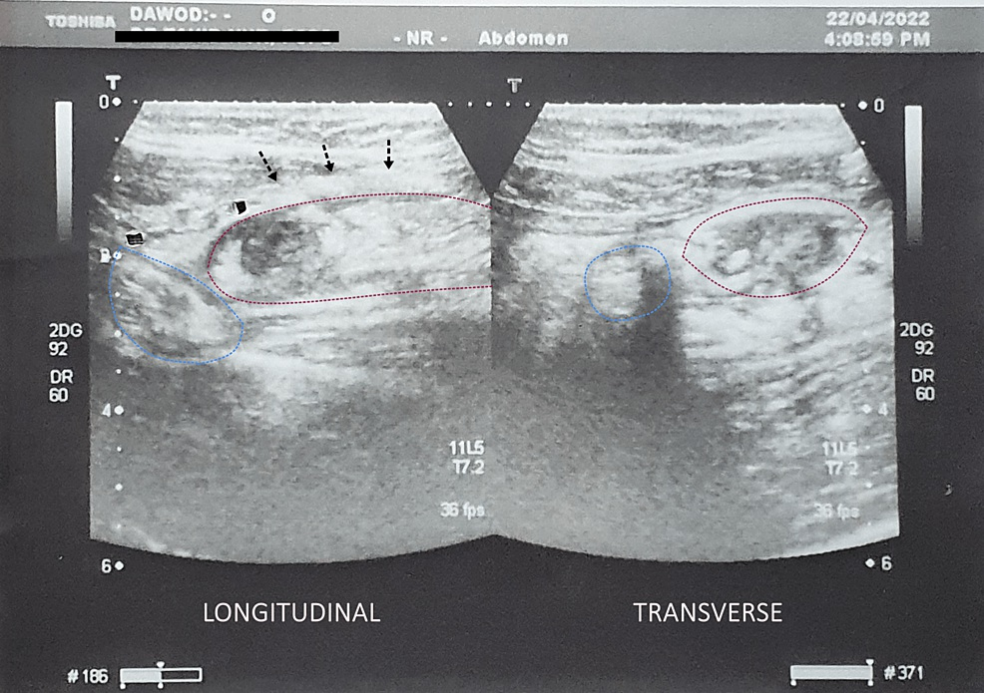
Initial abdominal ultrasound performed approximately 24 hours after the onset of symptoms. Longitudinal and transverse views reveal an edematous terminal ileum down to the ileocecal junction, a thickening of the ileocecal mesentery, and an inflamed appendicular stump. Dotted red line, terminal ileum; dotted blue line, appendicular stump; black arrows, ileocecal mesentery.
